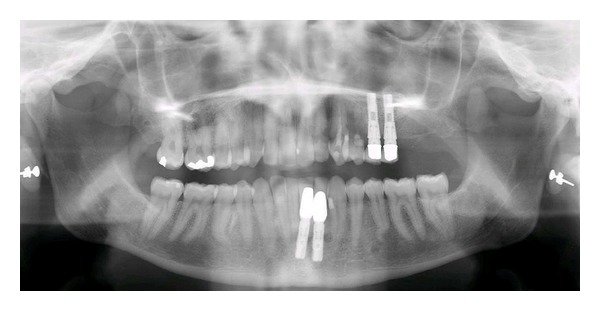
Patient 2: panoramic radiograph taken in 2007 demonstrating gradual resorption of the left-sided maxillary teeth when compared to the panoramic radiograph taken in 2004, demonstrated in Figure 8.
